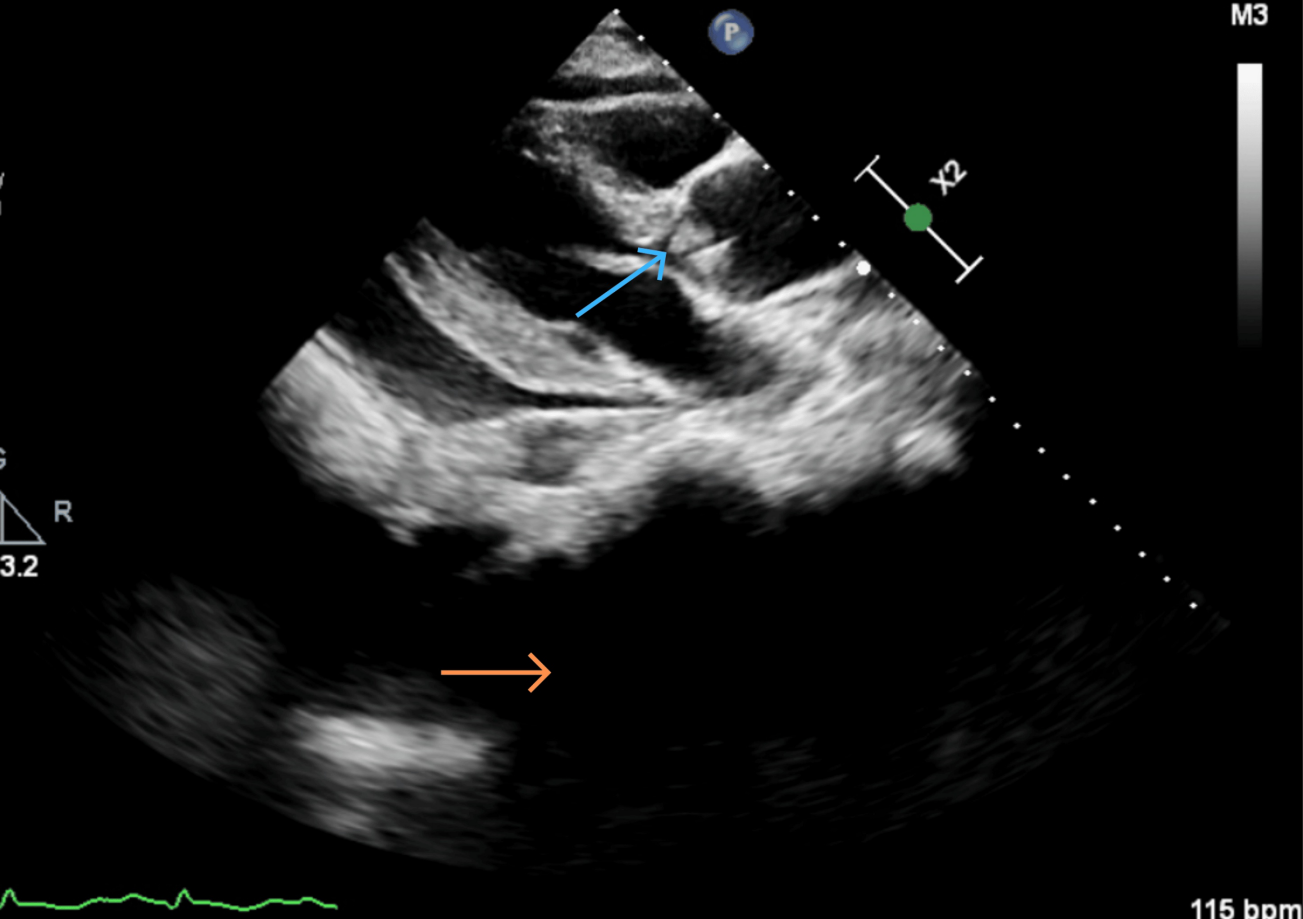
TTE PLAX view – pericardial effusion (orange arrow) and thick aortic valve (blue arrow) TTE: Transthoracic echocardiogram, PLAX: Parasternal long axis
